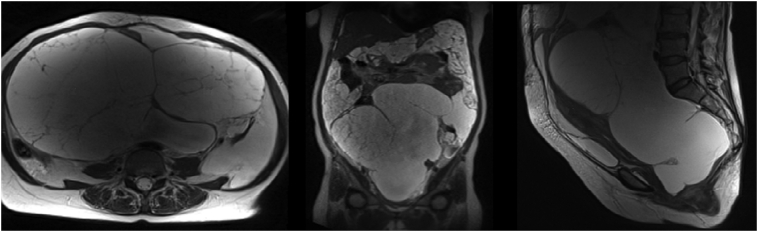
MRI whole abdomen showed accumulated fluid filling the entire intraperitoneal cavity and two multilocular cysts after six courses of chemotherapy.
